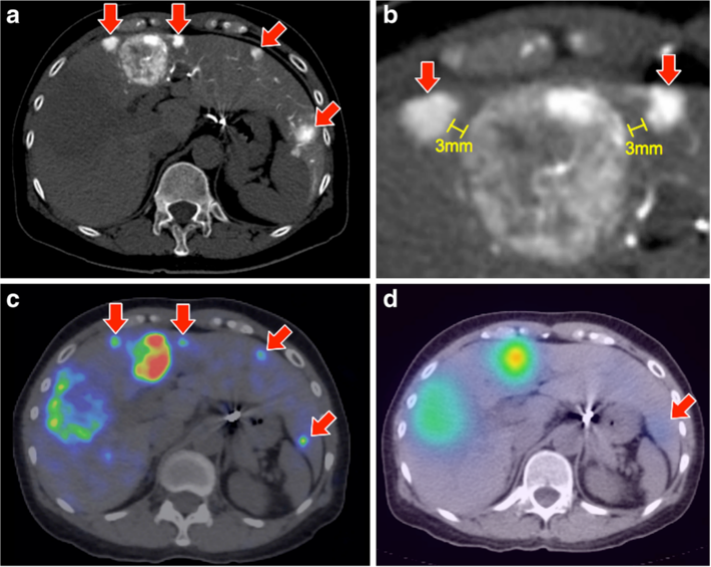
Patient 23. (a) Multifocal HCC seen on catheter-directed CT angiogram of the replaced left hepatic artery, arising from the left gastric artery. Four small tumors are indicated by arrows. (b) Enlarged view of segment IV shows the larger central tumor being flanked by two smaller, closely adjacent tumors separated by approximately 3 mm. (c) Post-radioembolization ^90^Y PET/CT confirms focal activity within the four small tumors, depicted in high resolution. (d)^90^Y bremsstrahlung SPECT/CT was barely able to detect subtle activity in the larger (arrow) of the four small tumors, but was unable to confirm any focal activity within the other three small tumors due to its low resolution.
